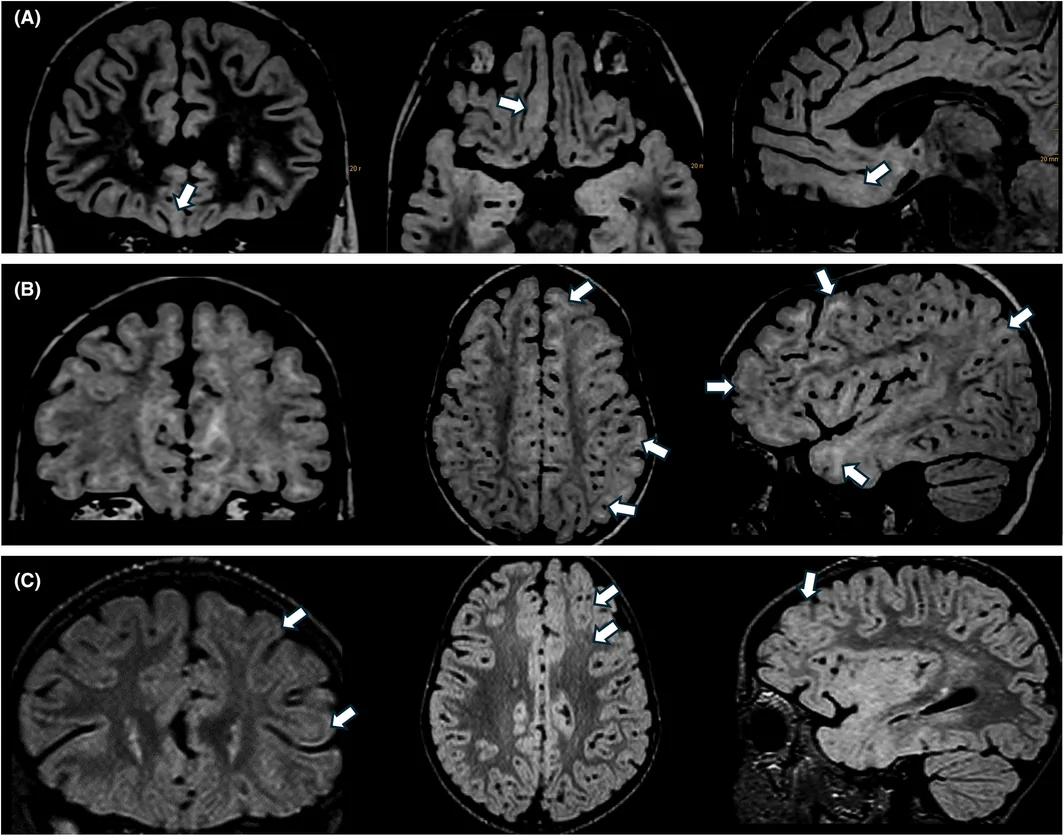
Representative magnetic resonance imaging (MRI) of individuals with mild malformation of cortical development with oligodendroglial hyperplasia in epilepsy. Representative MRI in coronal, axial, and sagittal planes demonstrates lesion extent and distribution. White arrows mark the lesions. (A) Circumscribed right orbitofrontal lesion. (B) Extensive left hemispheric lesion with an enhanced power button sign. (C) Left frontal lesion.
